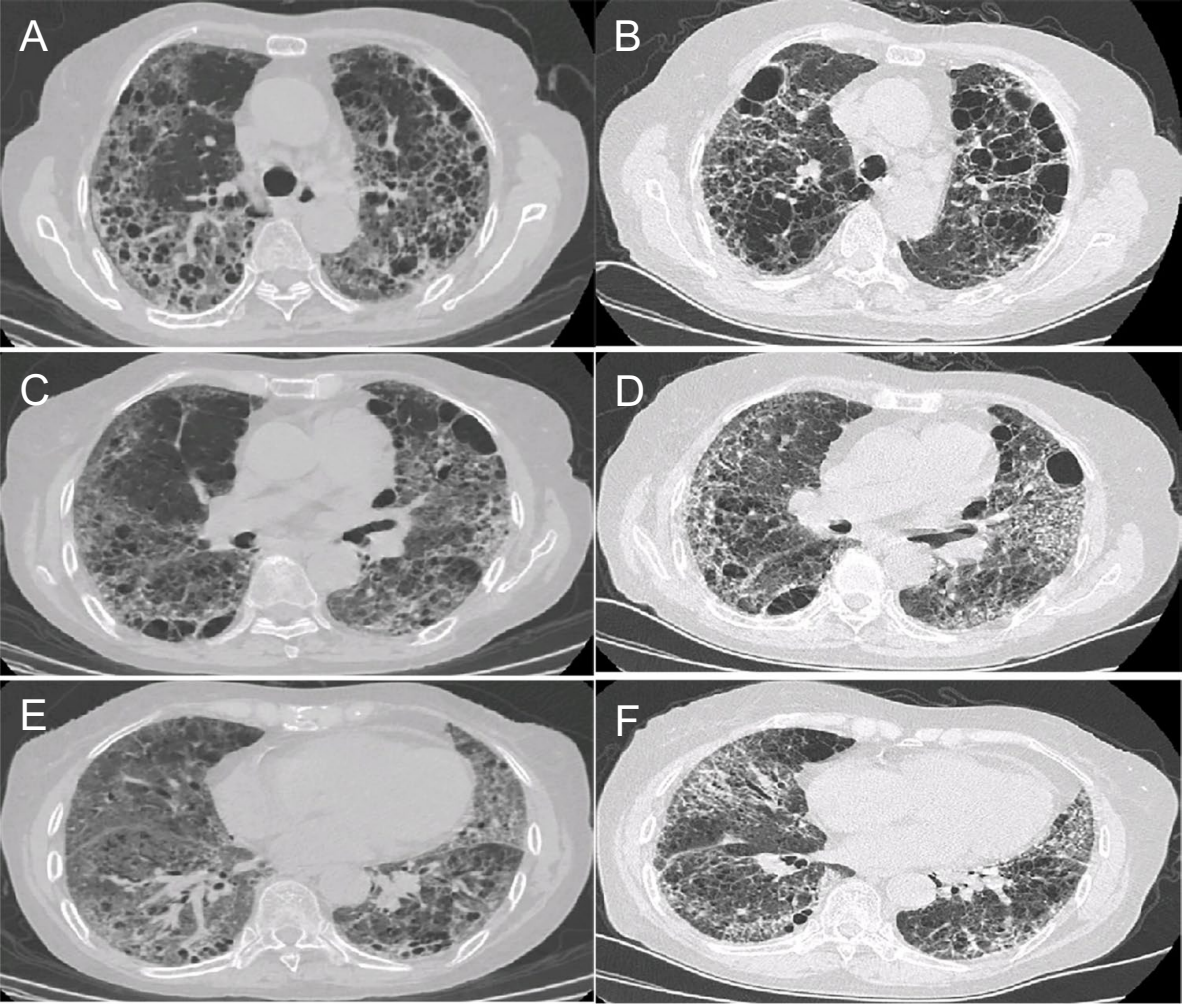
Progressive pulmonary fibrosis of unknown etiology. A 72-year-old female with anti-PL-12 antibody-positive dermatomyositis/interstitial lung disease. A, C, E High-resolution computed tomography (HRCT) images showing extensive honeycombing and cysts in the upper-middle lung and extensive ground-glass opacities and reticular abnormalities in the upper and lower lung. B, D, F HRCT images showing a substantial increase in the extent of reticular opacities and ground-glass opacities with traction bronchiectasis and increased lobar volume loss at the 7-month follow-up
